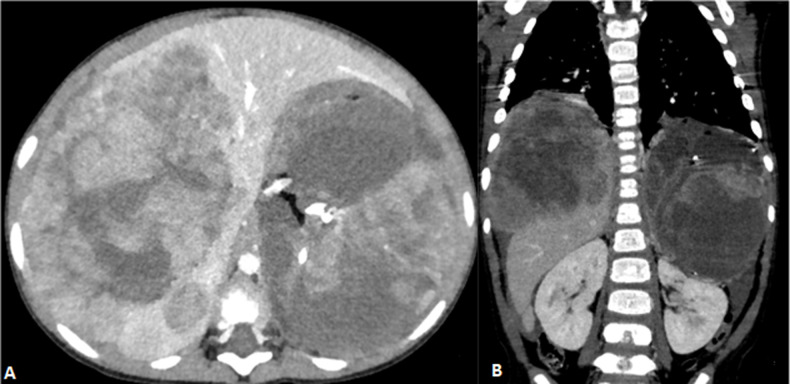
axial (A) and coronal CT (B) imaging showing a large left heterogeneous retroperitoneal mass with foci of calcification and fat. A large similar mass involving the VIII liver segment. Left kidney is displaced inferiorly and compressed against the posterior abdominal wall
